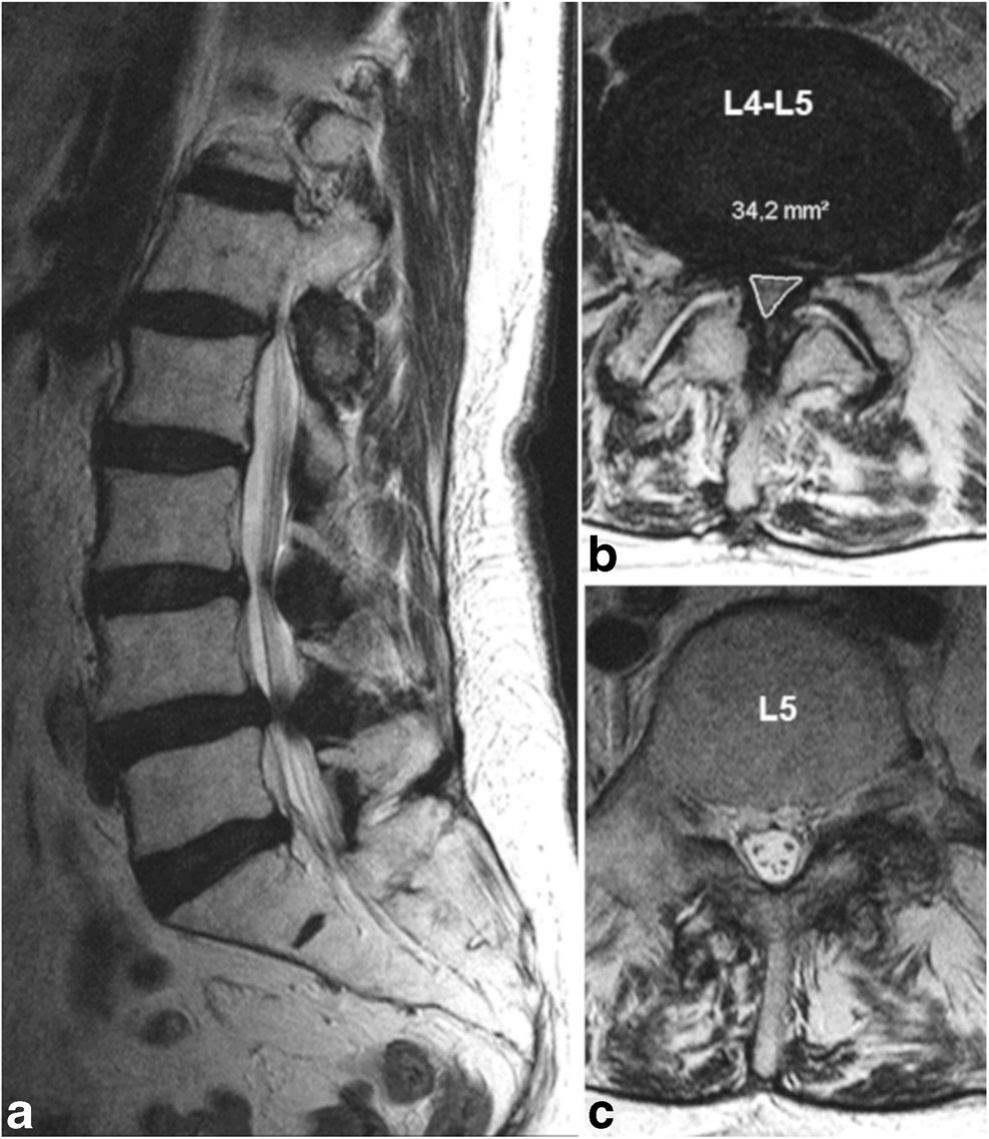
a Sagittal T2-weighted images (WI) with an almost normal course of the cauda nerve roots (CNRs) despite a stenotic level grade D at L4/L5 (b) according to Schizas et al. [17] in the axial slice. c The CNRs are distributed throughout the cross-sectional area of the dural sac (positive nerve roots sedimentation sign). No evidence of redundant nerve roots (RNR-)
